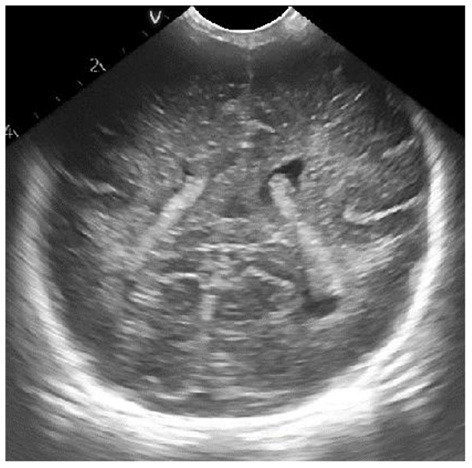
Ultrasonic image after adaptive median filtering.
